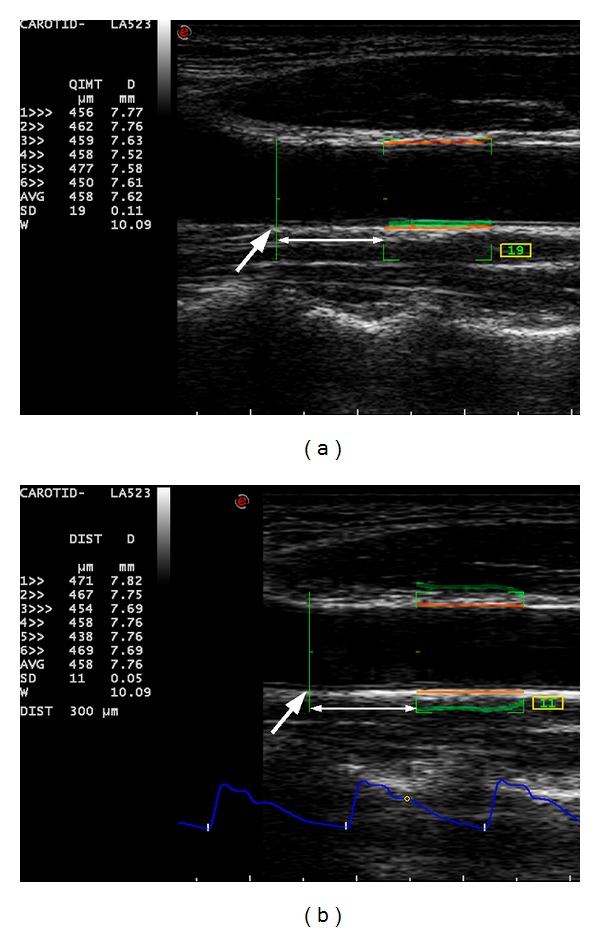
Measurements of the intima-media thickness and stiffness in the common carotid artery. (a) Longitudinal grey scale sonogram showing the measurement of the intima-media thickness of a common carotid artery (CCA) using radiofrequency-based quality intima-media thickness. (b) Longitudinal grey scale sonogram showing the measurement of the arterial stiffness of the same CCA using radiofrequency-based quality arterial stiffness. The arrows indicate the inferior end of the carotid bulb and the double-arrows lines show a distance of 1 cm.
